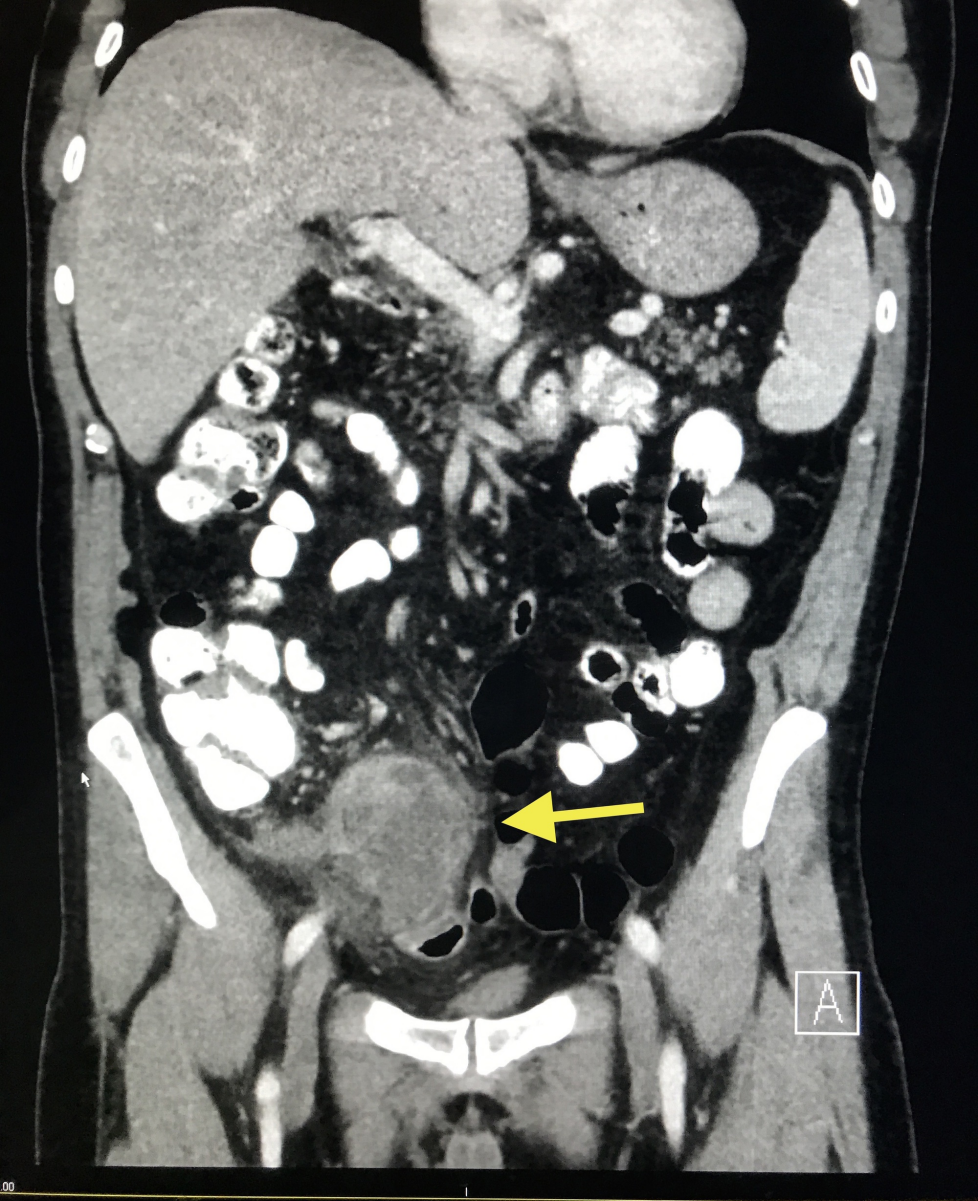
CT scan of the abdomen/pelvis showing perforated acute appendicitis with a 7-cm abscess and large phlegmonous change (yellow arrow) in the right lower quadrant with secondary inflammatory changes CT: computed tomography
